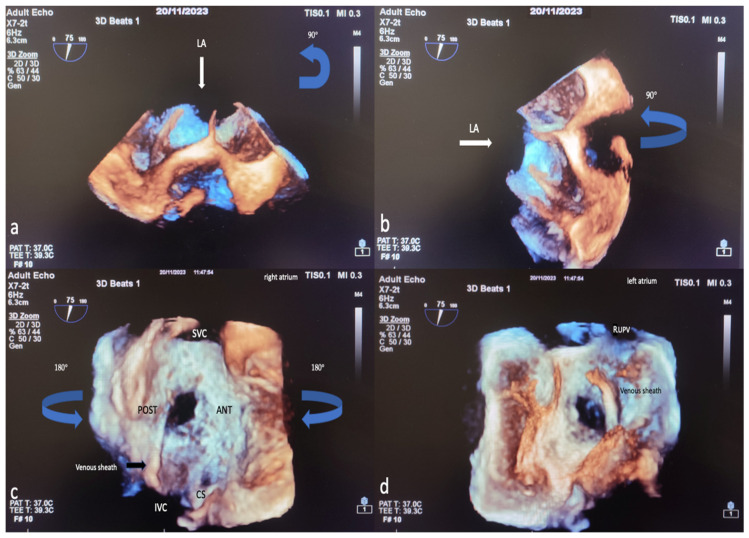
Image of percutaneous ASD closure process with 3D TEE, image optimization in 3D TEE. In 2D TEE examination, when the bicaval window (when examining the SVC-IVC plane section at approximately 80–120 degrees) is switched to 3D zoom examination, the Image on the monitor is obtained with the transducer facing the left atrium perpendicularly from above (a). The operator first views the Image 90 degrees counterclockwise (blue arrow) (a) in the sagittal axis and then rotates 90 degrees to the left on the vertical axis (blue arrow) (b) to obtain the right atrial view (c). After obtaining the RA (en face) view in this way, the LA (en face) view will be obtained by rotating 180 degrees in the vertical axis to the right or left (d). Based on these LA and RA facial views, ASD morphology and the status of the rims are easily identified. LA, left atrium; ANT, anterior; SVC, superior vena cava; POST, posterior; IVC, inferior vena cava; CS, coronary sinus; RUPV, right upper pulmonary vein; 2D, two-dimensional; 3D, three-dimensional. (The images included in this article were taken from Erzurum City Hospital Cardiology Clinic Echocardiography laboratory).
